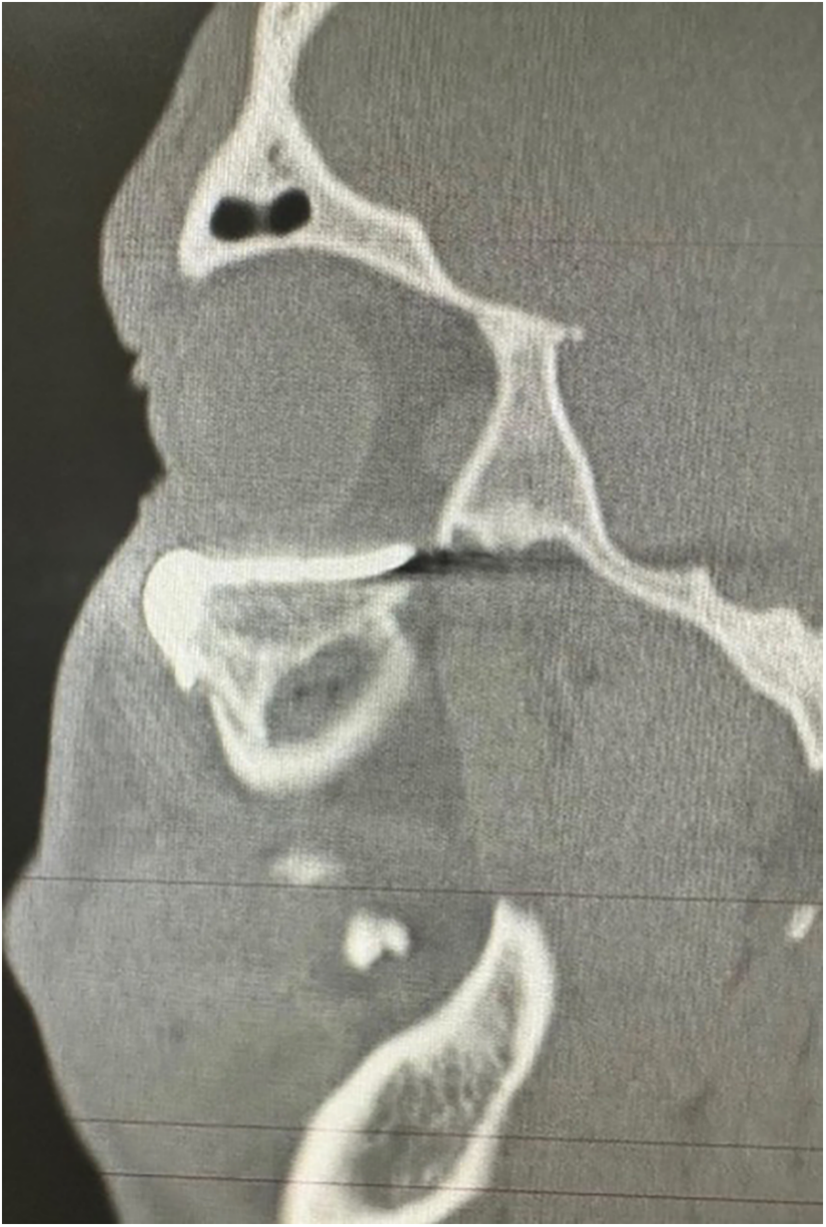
Sagital CT scan of the face showing the adaptation of the customized prosthesis.
